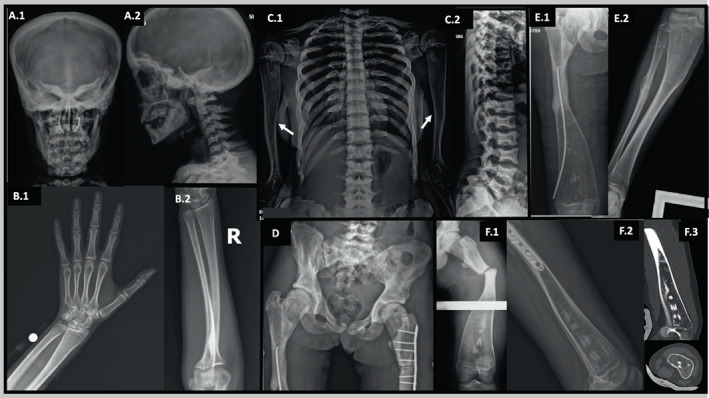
Patient 3. Radiographic findings at age 17 years. (*A.1*,*A.2*) Anteroposterior and lateral views of the skull show thick diploic space and diffuse osteosclerosis including the base, orbits with sphenoid wings, petrous bones, maxilla, mandible, and cervical spine. (*B.1*,*B.2*) Bones of the right hand and forearm are mildly undertubulated, but not osteosclerotic. (*C.1*,*C.2*) Osteosclerosis is marked in the wide ribs and present in portions of the clavicles. The vertebrae are sclerotic and flattened with coarse trabeculae and concavities of their superior and inferior margins are associated with disk expansion. Marked medullary cavity expansion distorts the proximal humeri showing cortical thinning and small focal metaphyseal osteosclerosis (arrows). (*D*) Anteroposterior view of the pelvis and proximal femurs shows diffuse patchy osteosclerosis and sclerotic rings paralleling the iliac crests, asymmetry, osteosclerotic femoral heads, wide femoral necks and trochanteric regions, and coxa valga. On the right, there is osteosclerosis and obliteration of the medullary cavity below the trochanters, a lateral cortical fracture line and intramedullary nail, and mild acetabular protrusio. On the left, a proximal femoral transverse fracture with plate‐and‐screw fixation lacks solid bridging, although new bone is medial to the fracture. (*E.1*,*E.2*) The right femur and tibia/fibula show marked expansion of the medullary cavities. The femur and tibia demonstrated cortical thinning at their ends and mid‐portion narrowing. The narrow mid femur has no medullary cavity. Bone atrophy has occurred proximal to the superior end of the femoral nail. A healing mid‐shaft fibular fracture is apparent. (*F*) The left femur shows marked medullary expansion and cortical thinning, focal areas of osteosclerosis, and an acute transverse fracture through its narrow mid portion (*F.1*), fixed internally with plate and screws (*F.2*). Computed tomography demonstrates the markedly thin cortex, expanded medullary cavity, and thin trabeculae (*F.3*).
